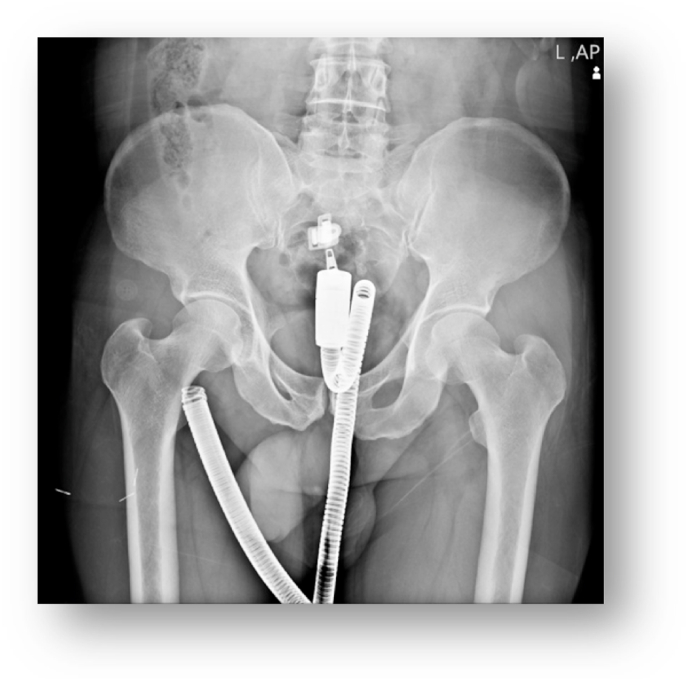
Intra-Rectal hose on abdominal plain film.
